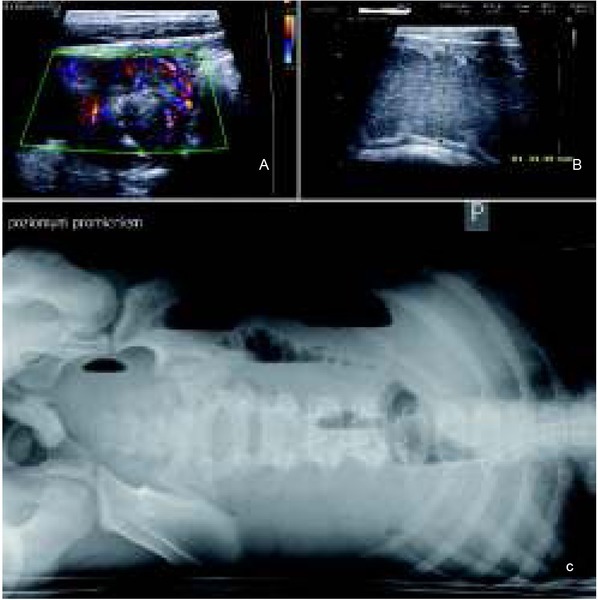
Imaging of the intramural tumor of the small intestine. A. Abdominal ultrasound before the onset of ileus, visible thickening of small intestine walls to 7 mm and increased vascularity in color Doppler study. B. Abdominal ultrasound at the time of malignant bowel obstruction - note a significant distention of the intestine. C. X-ray examination of the abdominal cavity in the supine position confirming bowel obstruction. Ryc. 1. Obrazowanie śródściennego guza jelita cienkiego. A. Obraz ultrasonograficzny jamy brzusznej przed wystąpieniem niedrożności jelit − wśród pętli jelitowych widoczne 7-milimetrowe zgrubienia ściany jelita cienkiego ze zwiększonym przepływem naczyniowym w badaniu kolorowym Dopplerem. B. Obraz ultrasonograficzny jamy brzusznej po wystąpieniu niedrożności przewodu pokarmowego - znaczne poszerzenie światła jelita cienkiego. C. Badanie rentgenowskie jamy brzusznej w pozycji leżącej - niedrożność jelit.
